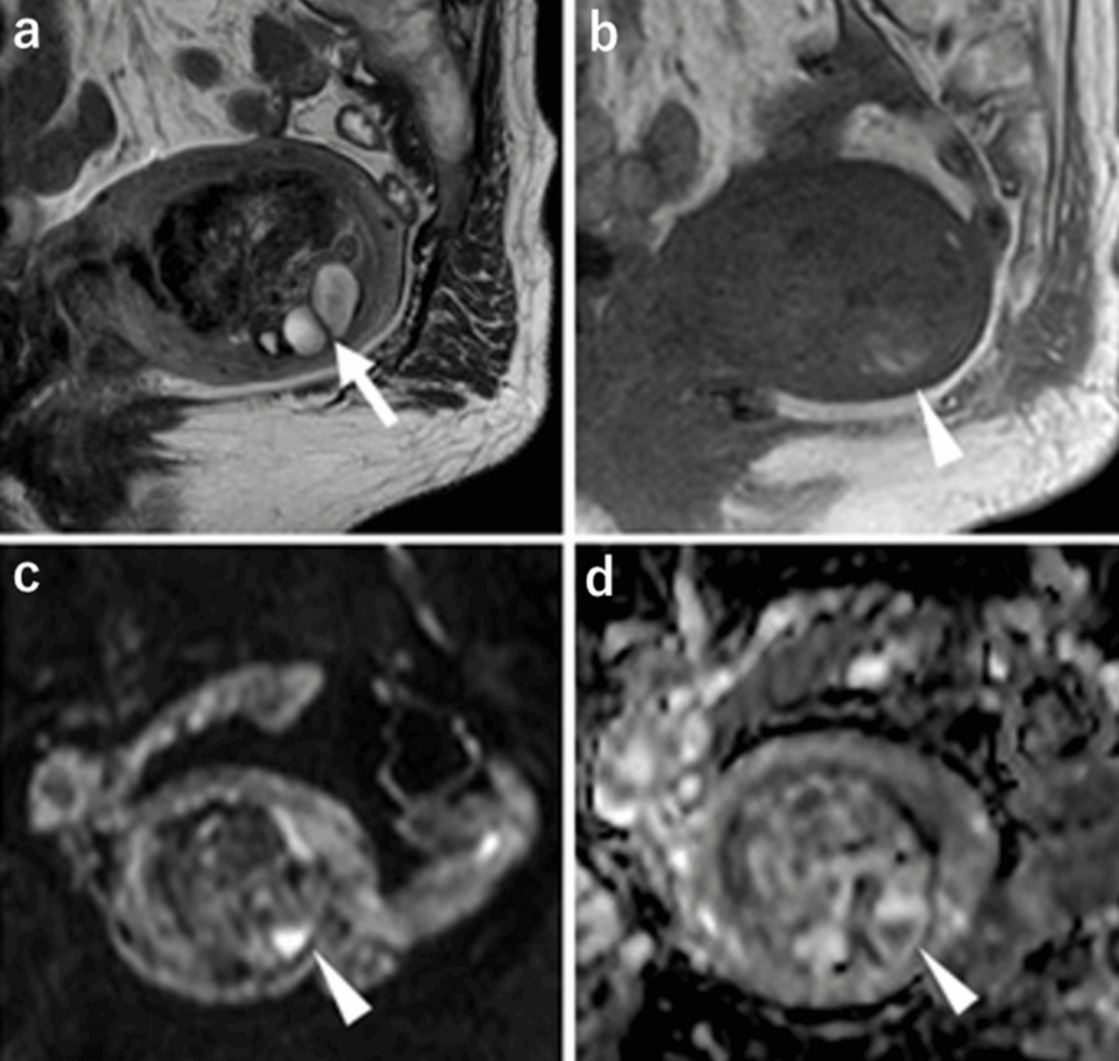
Pelvic MRI findings of the uterine mass (a) Sagittal T2-weighted image showing a mass occupying the uterine cavity, demonstrating heterogeneity and hypointensity relative to the myometrium, with multiple hyperintense foci indicative of cysts (arrow). (b) Sagittal T1-weighted image showing an isointense mass relative to the myometrium with small hyperintense foci suggestive of a hemorrhage (arrowhead). (c, d) Axial diffusion-weighted imaging with a b-value of 1000 s/mm^2^. (c) The solid components in the tumor demonstrate low signal intensity with high apparent diffusion coefficient values (d), except for the areas of hemorrhage (arrowhead). MRI: magnetic resonance imaging
